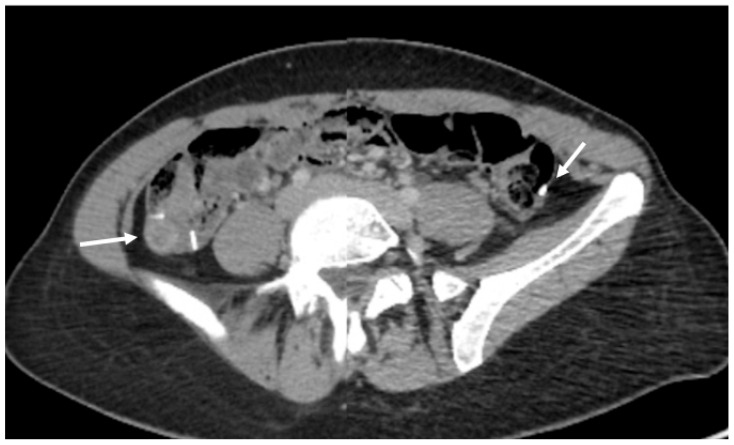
Postoperative pelvic MRI showing radiopaque ovarian clips. Pelvic MRI demonstrates radiopaque clips (white arrows) placed on each ovary (two on the right side and one on the left) for postoperative localization.
